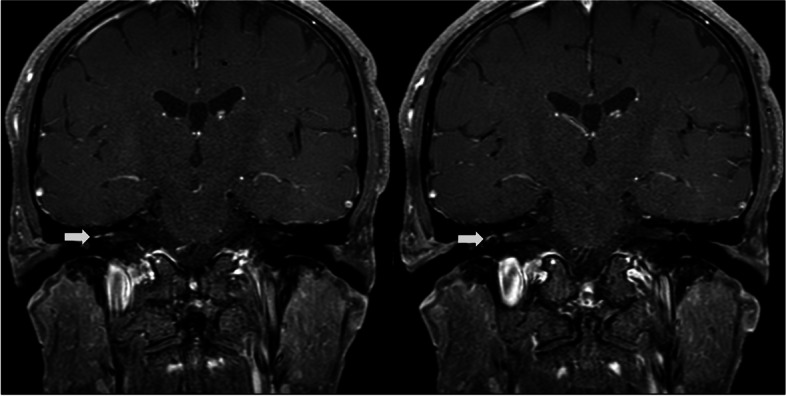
Brain magnetic resonance image of patient 2. Contrast-enhanced T1-weighted image reveals enhancement in the right facial nerve (White arrows)
